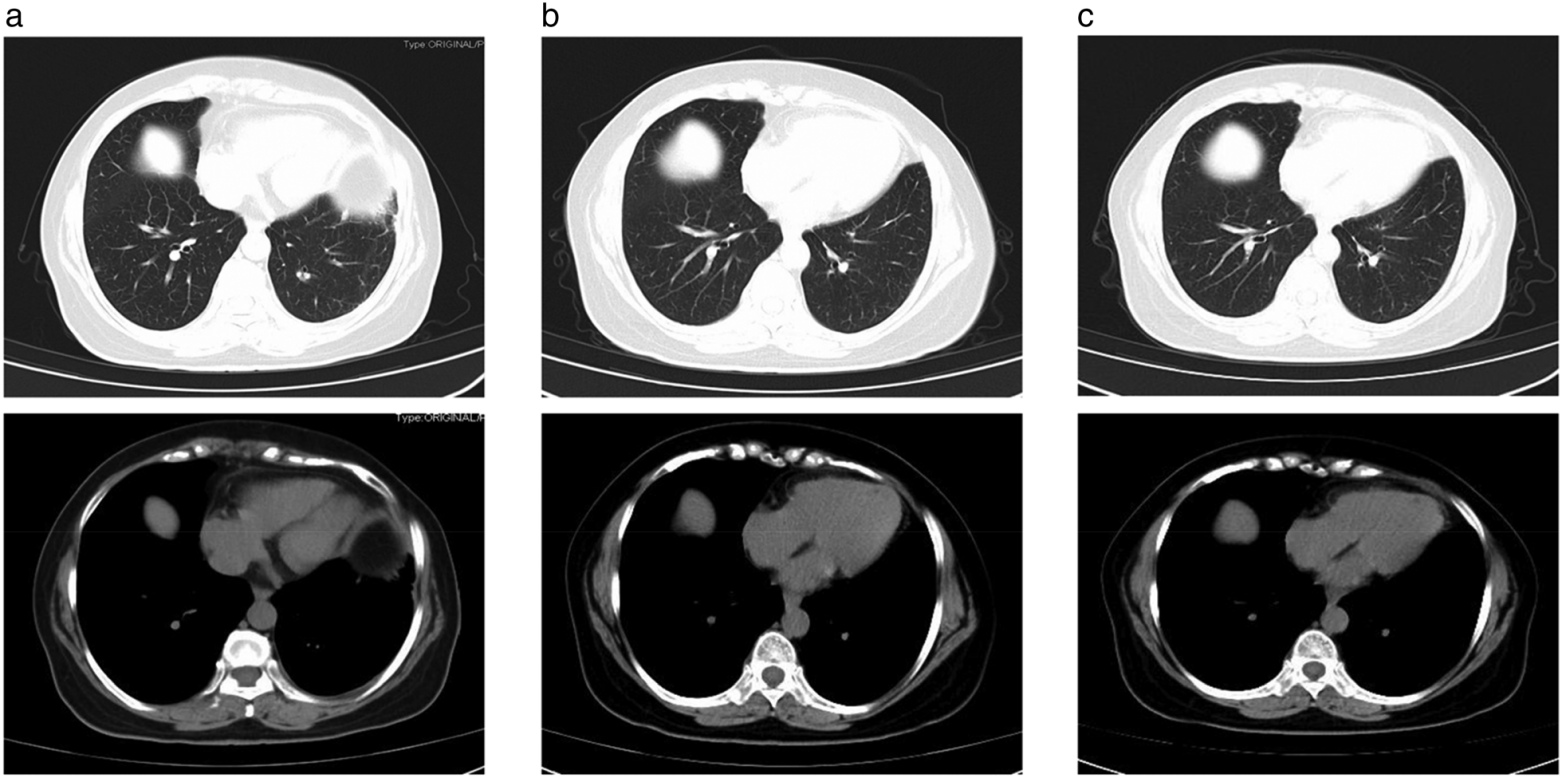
Subsequent chest CT scans performed (a) two, (b) seven and (c) 10 months after initiation of crizotinib treatment, and no signs of tumor recurrence were evident.
